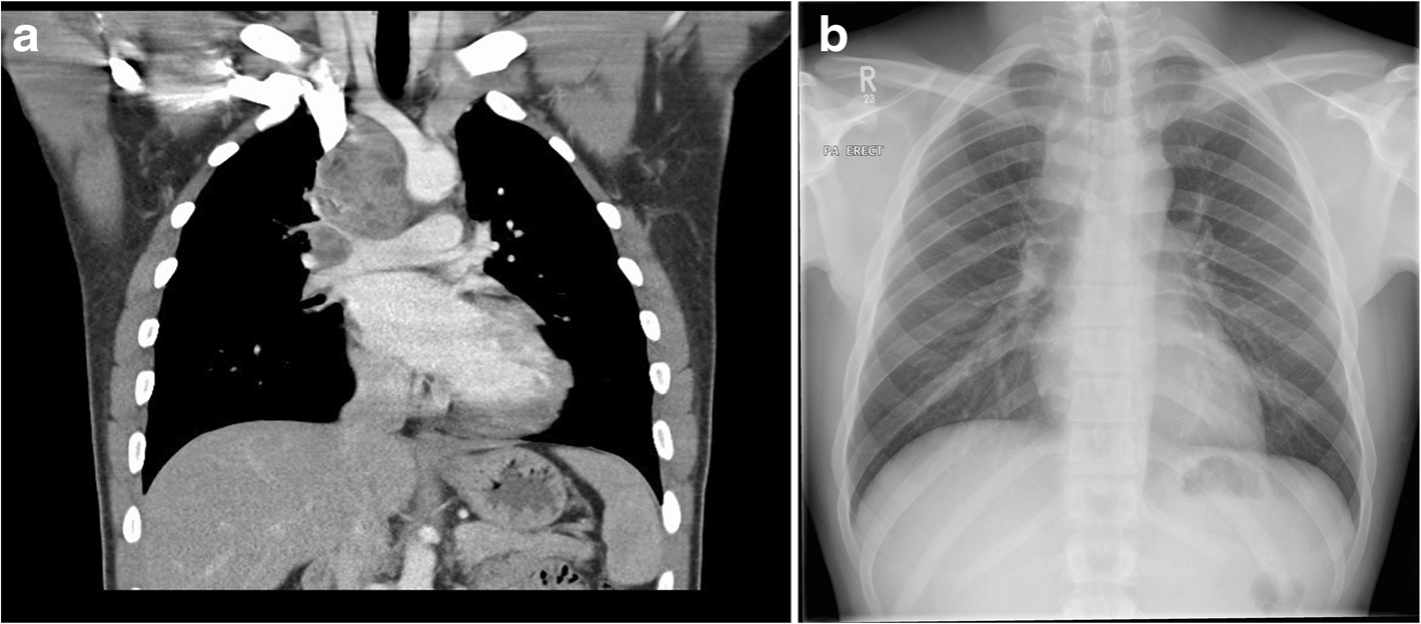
a Initial chest x-ray demonstrates soft tissue enlargement within the right paratracheal stripe; b Contrast computed tomography of the thorax shows a large right-sided mediastinal mass with accompanying lymphadenopathy encompassing the pre-tracheal, para-tracheal, tracheobronchial, hilar and subcarinal regions. (The images have been placed in reverse order and so the caption does not match the image. The CXR should be Figure 1a and the CT image should be Figure 1b)
